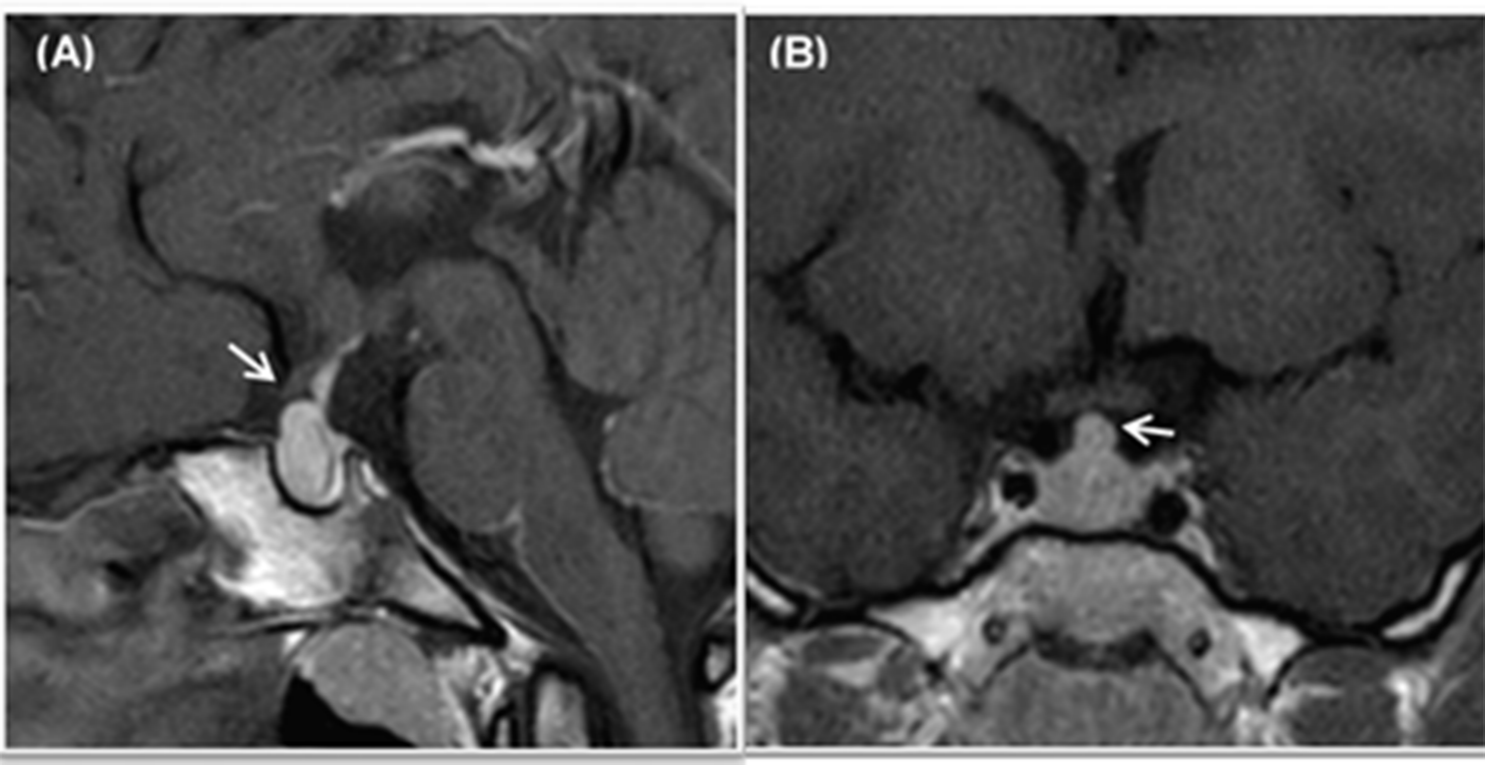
Pituitary gland Magnetic Resonance Imaging (MRI) after Gadolinium-DTPA administration of a patient at the time of diagnosis of pituitary hyperplasia and severe hypothyroidism. Sagittal (a) and Coronal T1-weighted images (b) that showed diffuse enlargement of the pituitary gland and a homogeneous enhancement of the gland. The enlarged pars tuberalis extended into the suprasellar cystern with mild compression of the optic chiasm (arrows)
